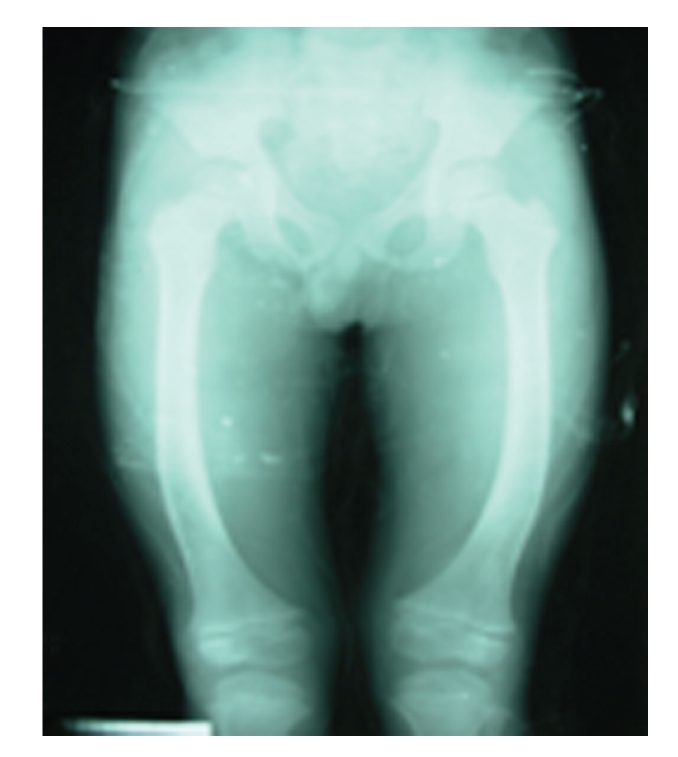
X-ray for another 7-year old boy.
